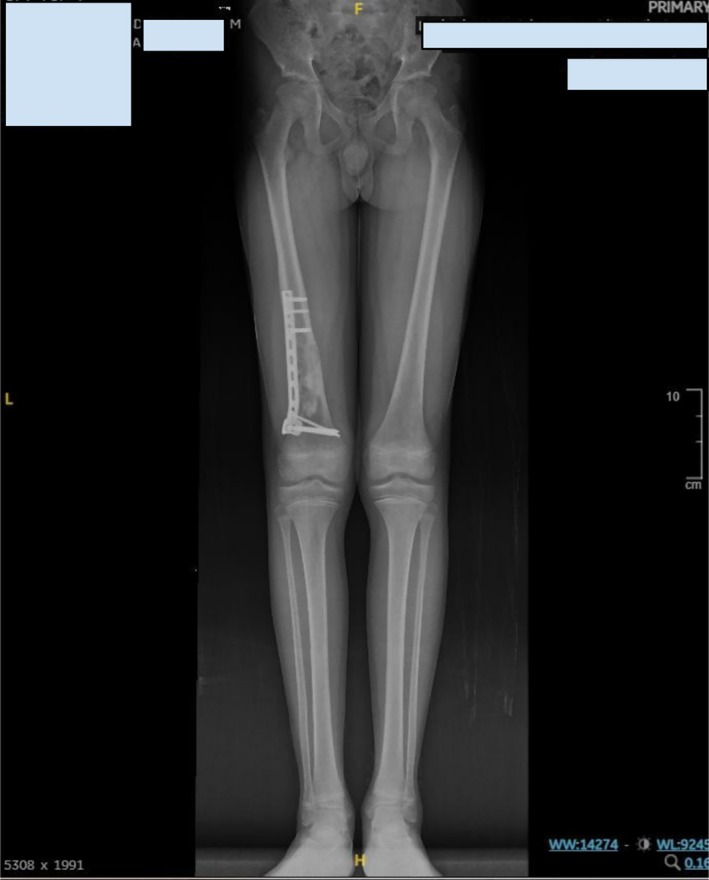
Nine‐month follow‐up standing long‐leg radiograph demonstrating complete healing and equal limb length. The distal femur shows complete radiographic healing with maintained alignment and an intact plate‐and‐screw construct. Comparison of both lower limbs demonstrates no clinically significant leg‐length discrepancy.
